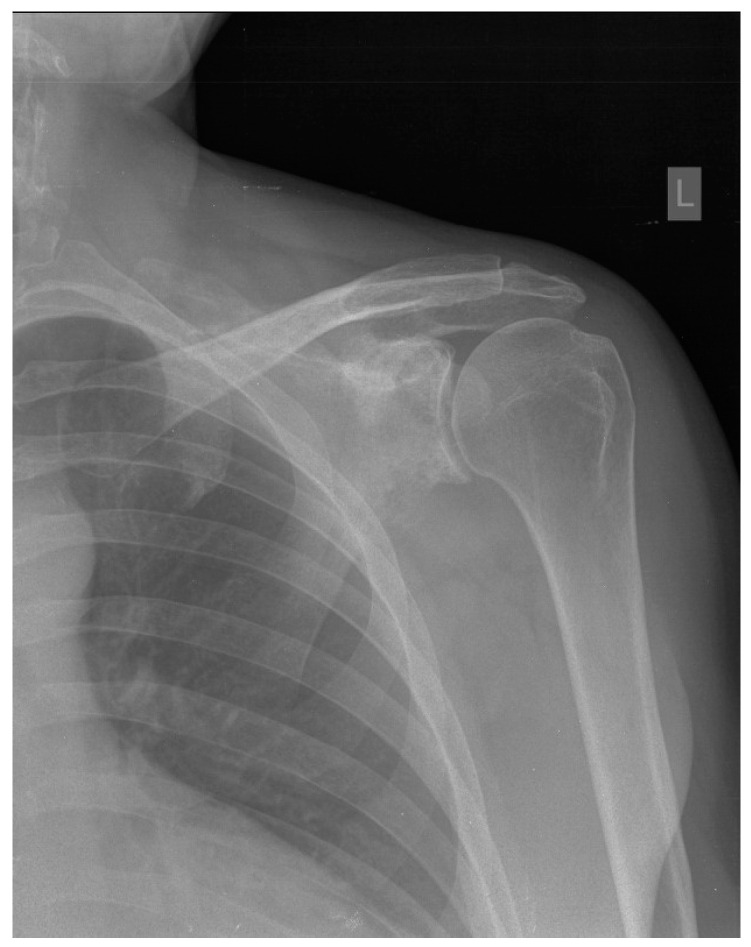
X-rays, anteroposterior (AP) view of the left shoulder. Multiple areas of decreased bone mineralization and multifocal osteolytic lesions are seen in the left scapula, with an area of breached cortical bone in the inferior part of the scapular neck.
